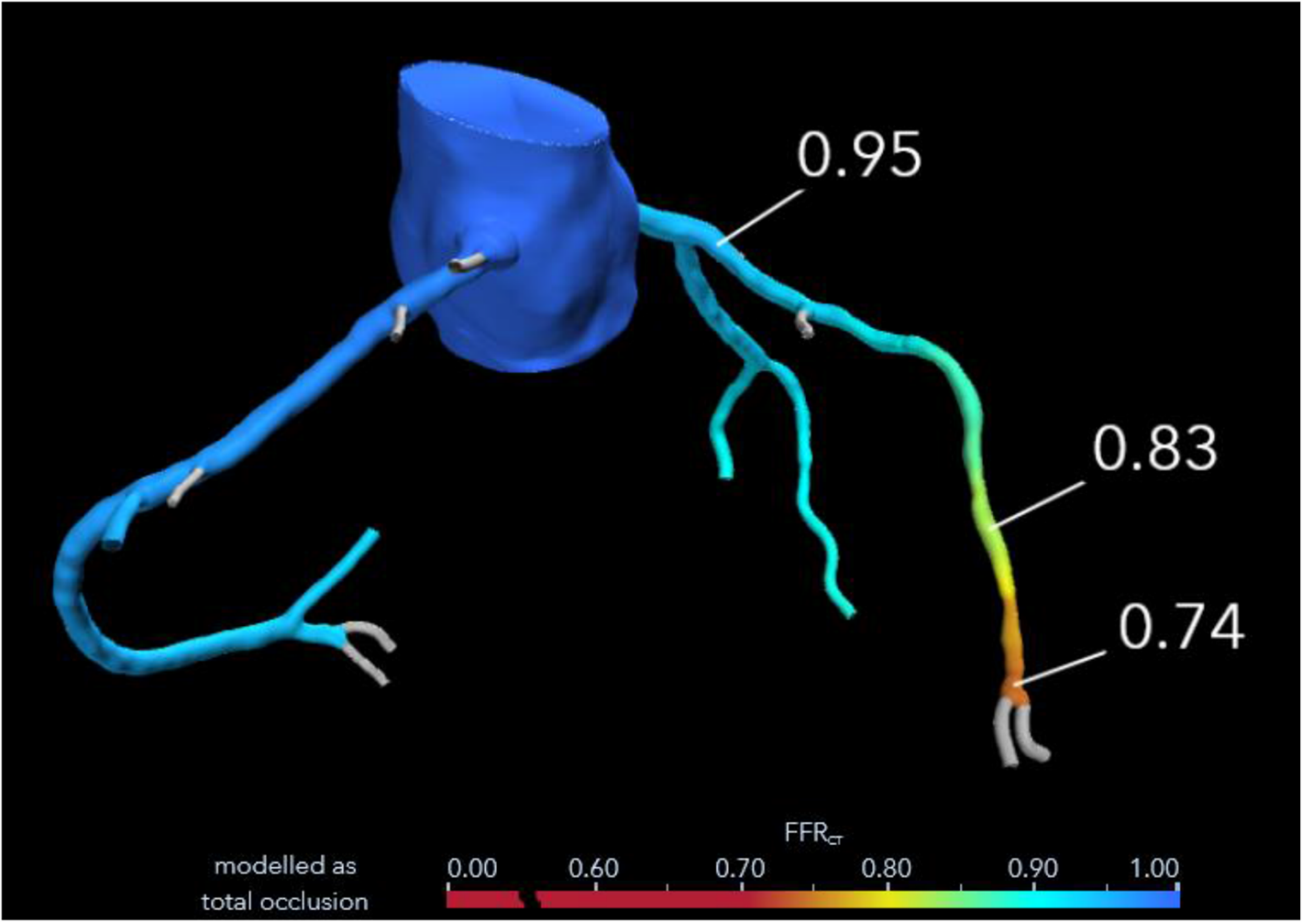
CT coronary angiogram with CT-FFR indicates distal left anterior descending coronary artery (LAD) ischemia. Right anterior oblique-cranial projection
